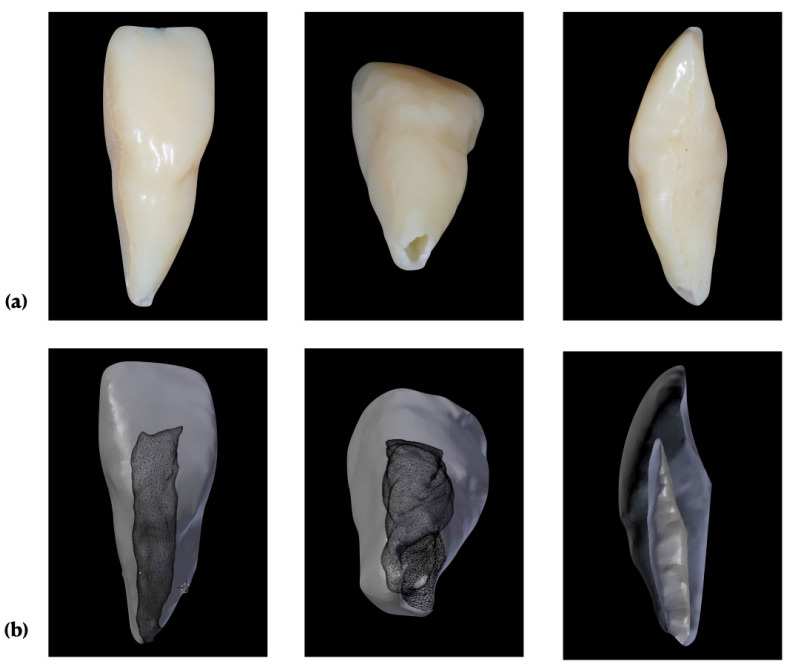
(a) 3D-printed central maxillary incisor, view from buccal, apical, and longitudinal section; (b) 3D rendering based on a micro-computed tomography imaging.
